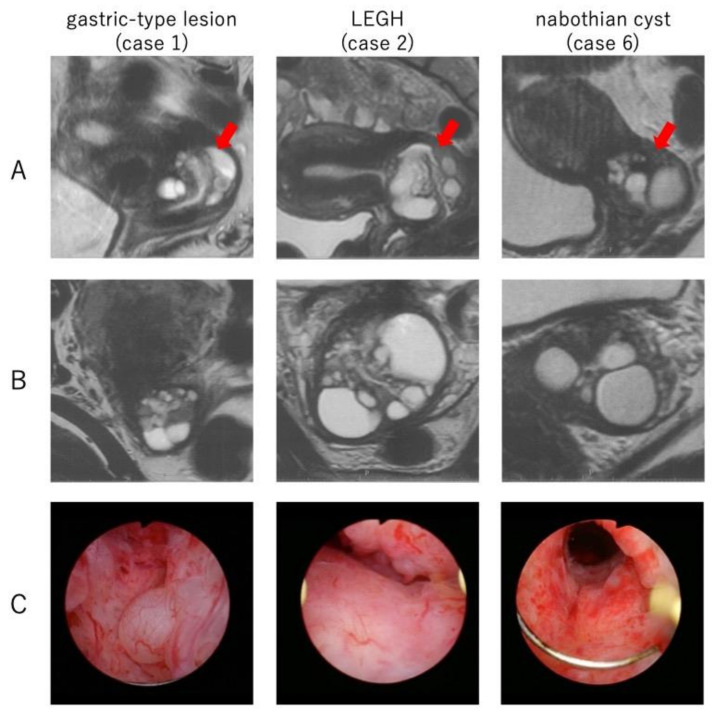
MRI of the uterine cervix, and images of the lesions by hysteroscopic observation. (A) T2-weighted image, sagittal section. Cystic lesions in the cervix are stretching to the internal os of the uterus (arrows). (B) T2-weighted image, horizontal section. The sizes of the lesions and cysts differ among cases. (C) Cervical cystic lesions observed from inside the cervix by a hysteroscope.
